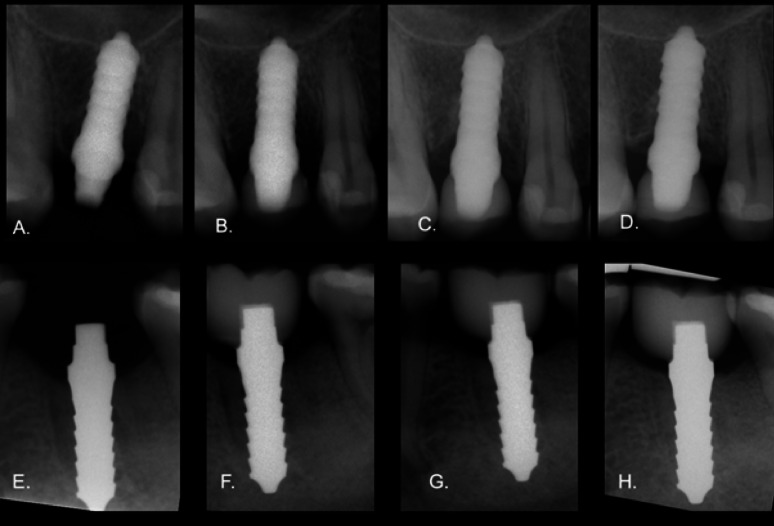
Intraoral radiographs of a one-piece titanium implant placed in tooth 14 in a 25 year-old female patient, taken directly after implantation (A), at baseline (B), 6 months (C), and 36 months (D) after implant placement. Additionally, a zirconia implant is placed in tooth 36 in a 34-year-old female patient, taken directly after implantation (E), at baseline (F), 12 months (G), and 72 months (H) after implant placement
